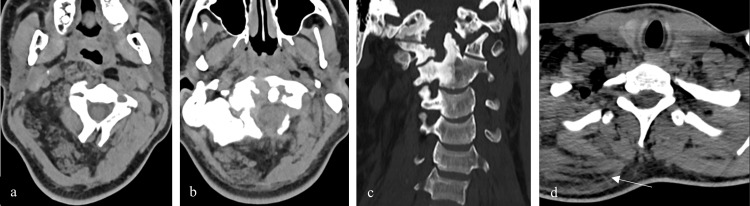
Cervical CT with axial images in soft window (a) (b) (d) and coronal reformation in bone window (c) demonstrates a diffuse lipomatous infiltration of the right suboccipital, posterior paravertebral, and prevertebral muscles, as well as the ipsilateral posterior cervical, retropharyngeal, and carotid spaces, with increased volume of these regions, deforming the posterior wall of nasopharynx and oropharynx (a). It reveals a periodontoid soft tissue mass (b), with remodeling of the right lateral mass of C2, the odontoid process and the anterior arch of C1 (c). The bone abnormalities were well delimited and exhibited cortical, subchondral and medullary sclerosis (c). Diffuse lipomatosis of the muscles and spaces of the right scapular region was also observed (arrow) (d).
